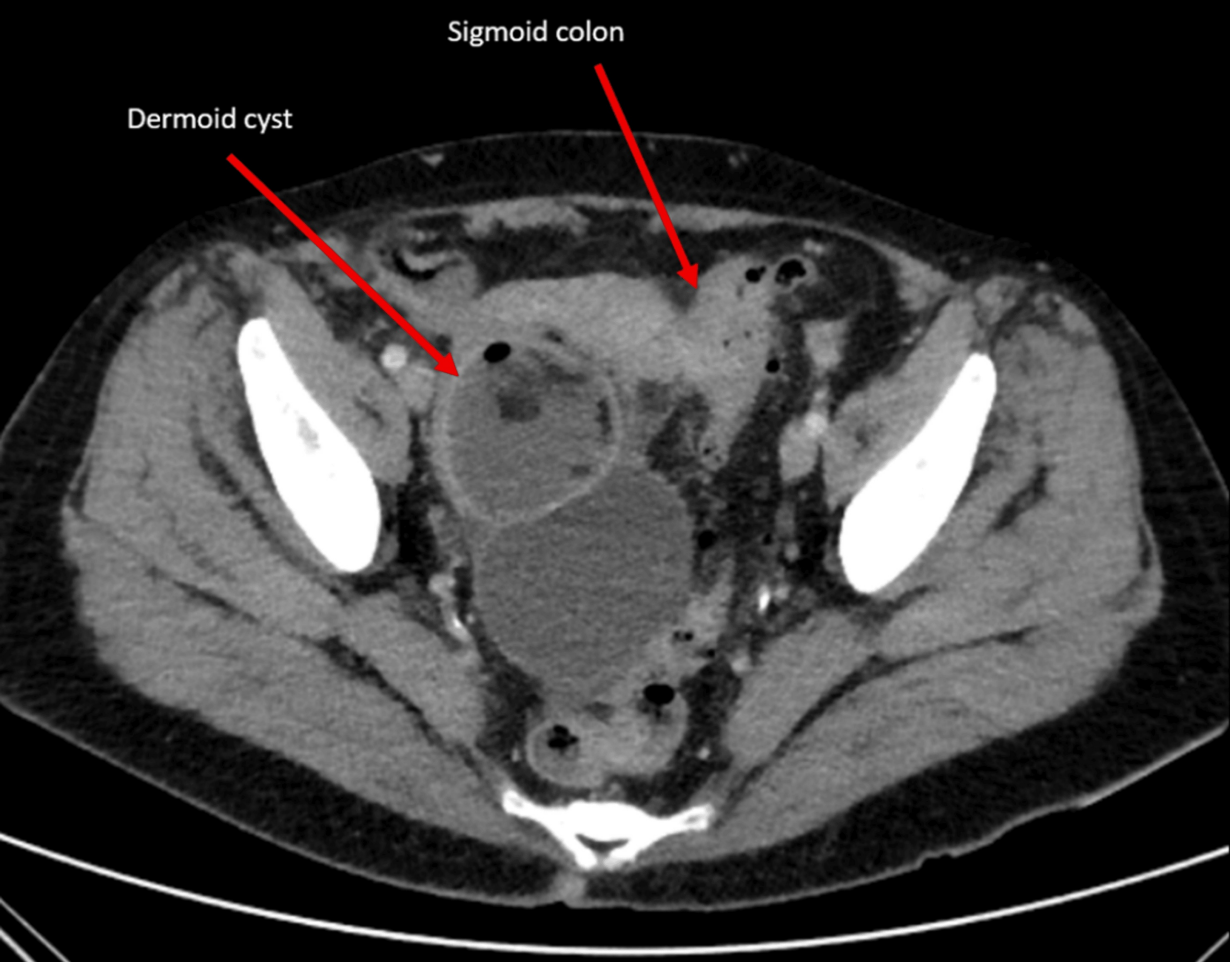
Axial portal venous contrast computed tomography scan demonstrating an inflamed sigmoid colon and fistulous communication with large multiloculate dermoid cysts (red arrows). Gas locules can be seen within the dermoid cyst.
